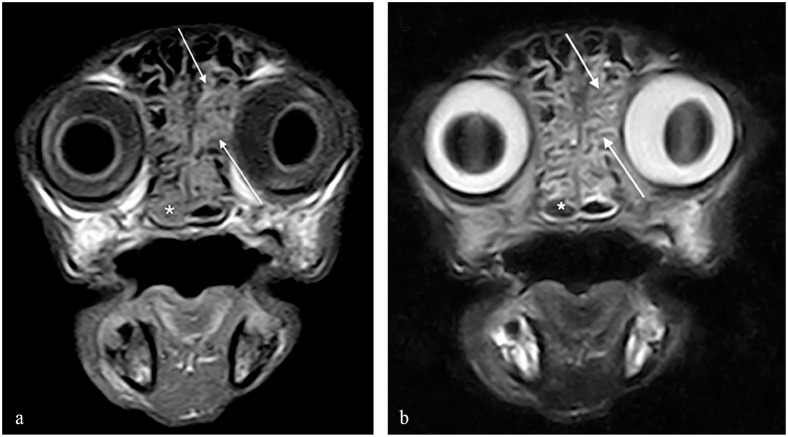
Transverse MRI scans at the level of the caudal nasal turbinates. (a) A T1-weighted image is provided as well as (b) a T2-weighted image. Material is visible between the turbinates (arrows) and in the right choana (asterisk) in the normally gas-filled (signal void) space
